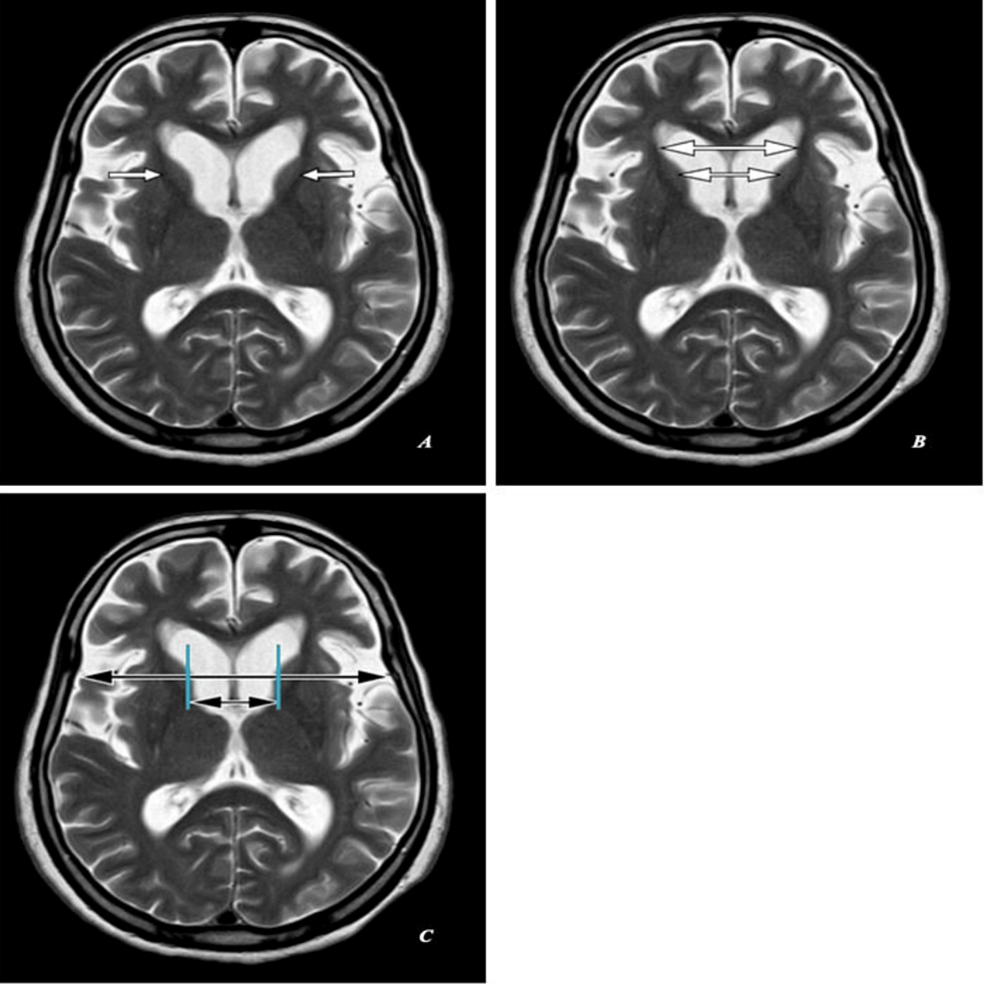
Magnetic resonance imaging (MRI) brain (T2-weighted): (A,B,C) Axial sections of the same patient. (A) Shows head of bilateral caudate nuclei mild atrophy with ex-vacuo mild dilatation of frontal horns of lateral ventricles (short white arrows). (B) Shows frontal horn width (large double white arrow) to intercaudate distance (small double white arrow) ratio (FH/CC) of 1.6, mildly decreased. (C) Shows an intercaudate distance (small double black arrow) to inner table width (large double black arrow) ratio (CC/IT) of 0.24, mildly increased.
